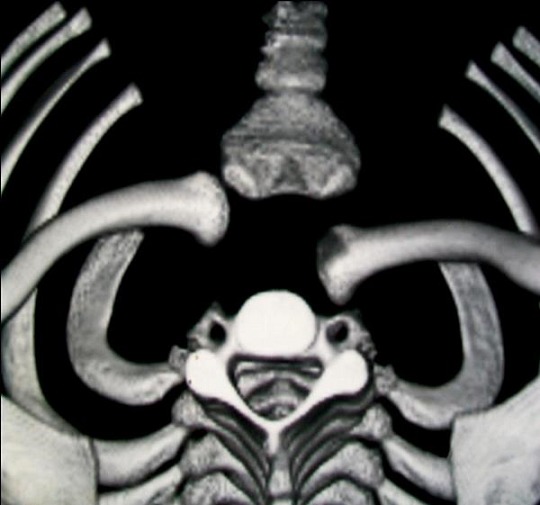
Chest CT scan showing right posterior sternoclavicular joint dislocation
